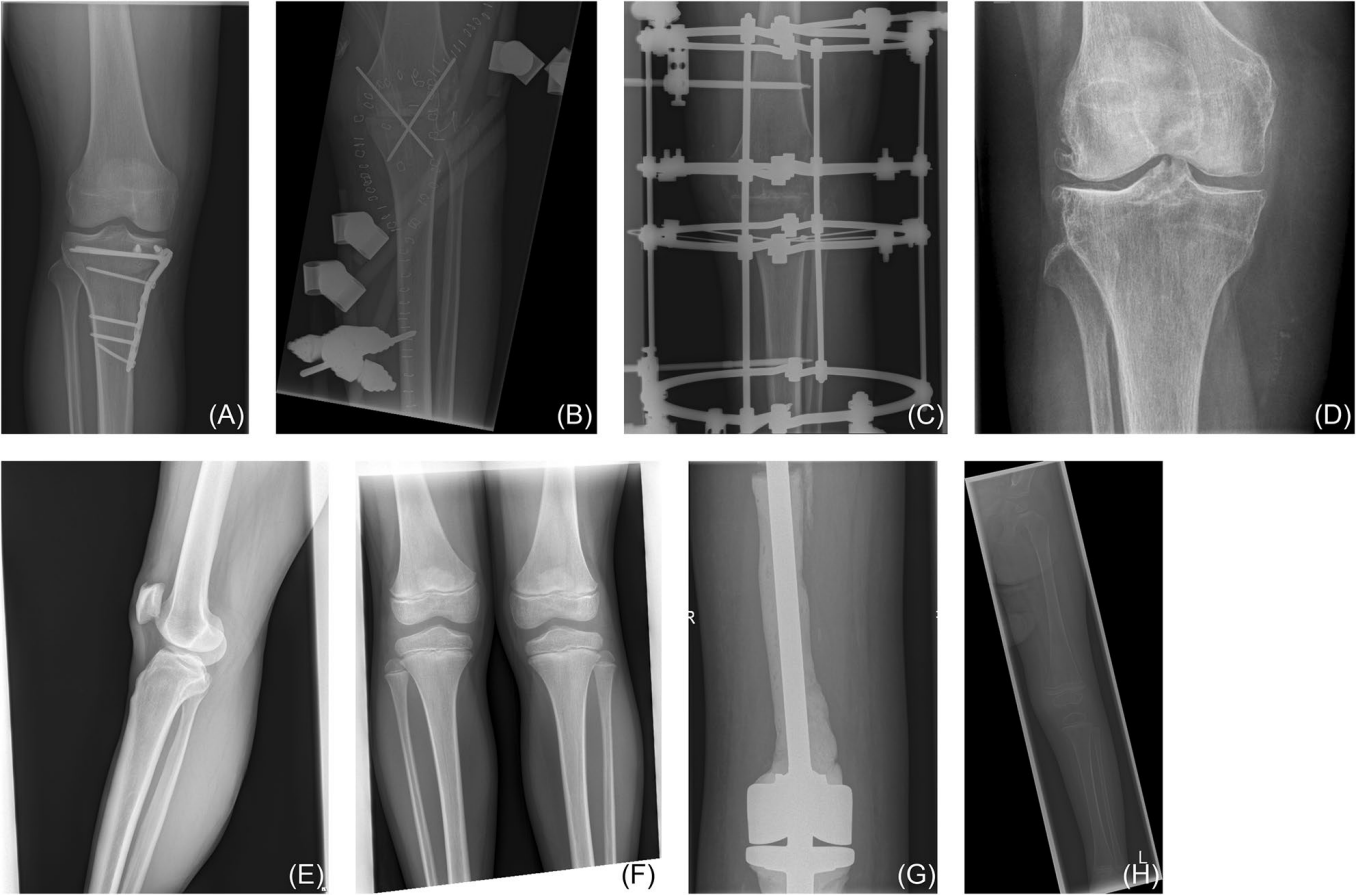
Knee radiographs for nine patients. The upper row depicts radiographs that were included into the study (images were cropped to remove the anatomic side markers) while the lower row shows examples of radiographs that were excluded. (A) Male patient (40.1 years) (B) Male patient (34.4 years) (C) Male patient (34.5 years) (D) Female patient (76.2 years) (E) Male patient (61.2 years), excluded because of lateral view (F) Male patient (11.1 years) excluded because of both knee are visible (G) Female patient (73.2 years) excluded because of knee is not fully visible (H) Female patient (1.9 years) excluded because of low image quality.
